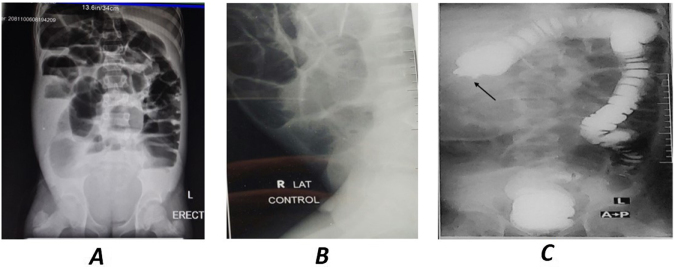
Dilated and edematous Small bowel loops + airfluid levels. (A) Plain abdominal X-ray (control). PA erect view. (B) PA lateral view. (C) Barium Enema Abd X Ray (AP view). Abrupt termination and narrowing of hepatic flexure, giving the “apple core” deformity.
